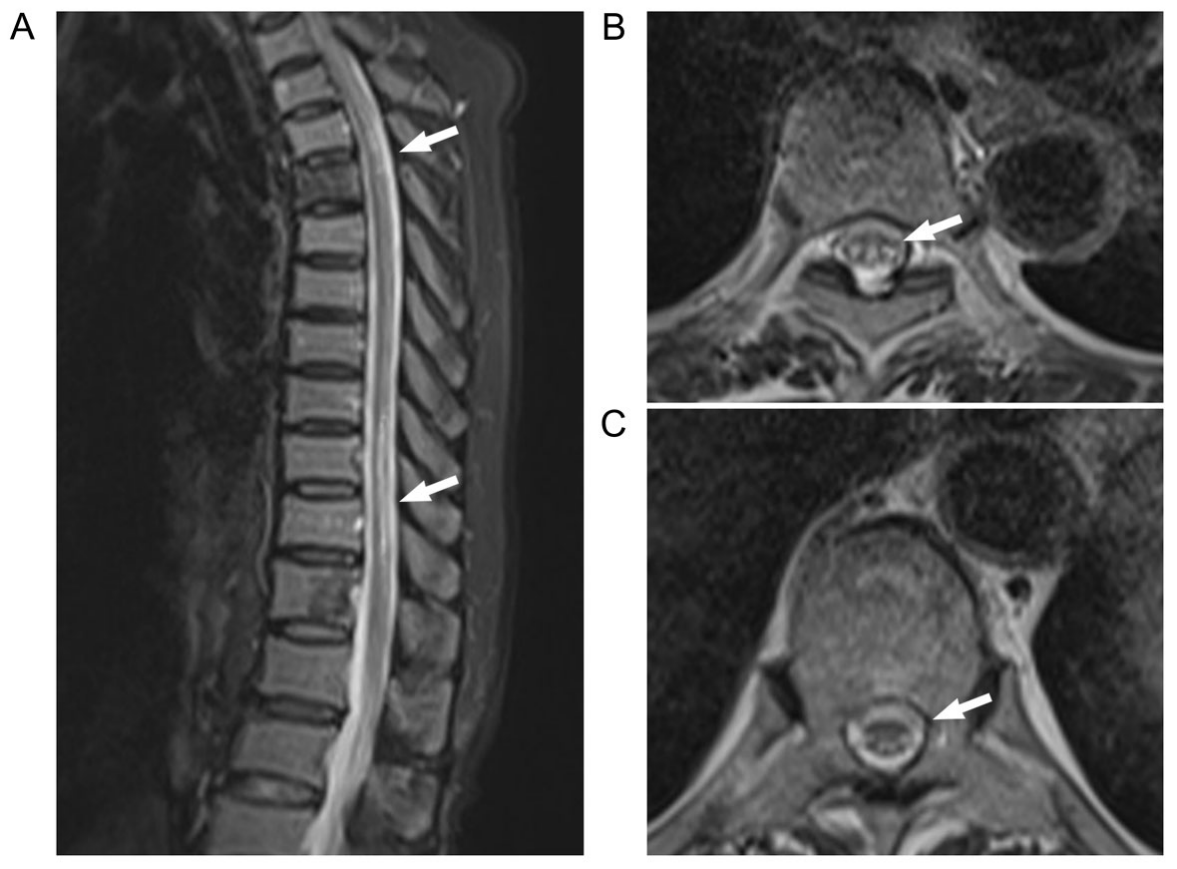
T_2_-weighted magnetic resonance images of thoracic vertebra in Case 2. A, Sagittal view shows high-signal intensity lesions in the thoracic spinal cord (arrows). B and C, Axial views show the spinal cord “small print” sign at different positions in the thoracic segment (B, T5 level; C, T10-T12 level; arrows).
